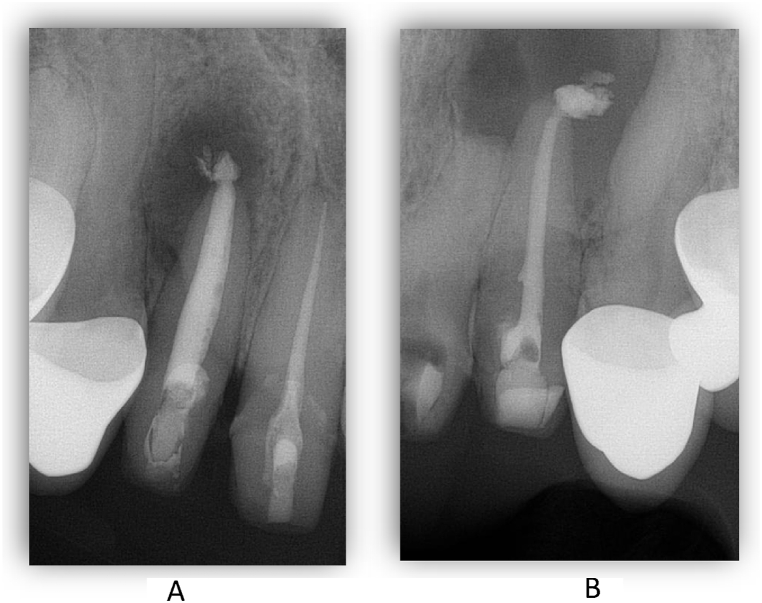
Periapical X-rays after the rootcanal treatments. 12 and 11 teeth (A) and the 22 tooth (B).
